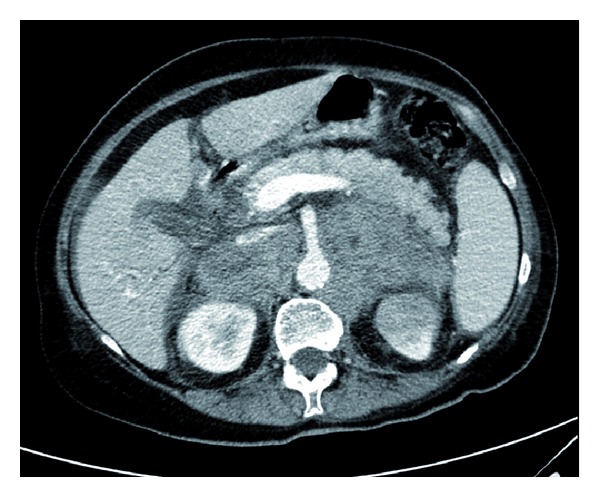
Abdominal CT scan showing large bilateral adrenal masses of homogenous appearance. No other pathology was demonstrated.
